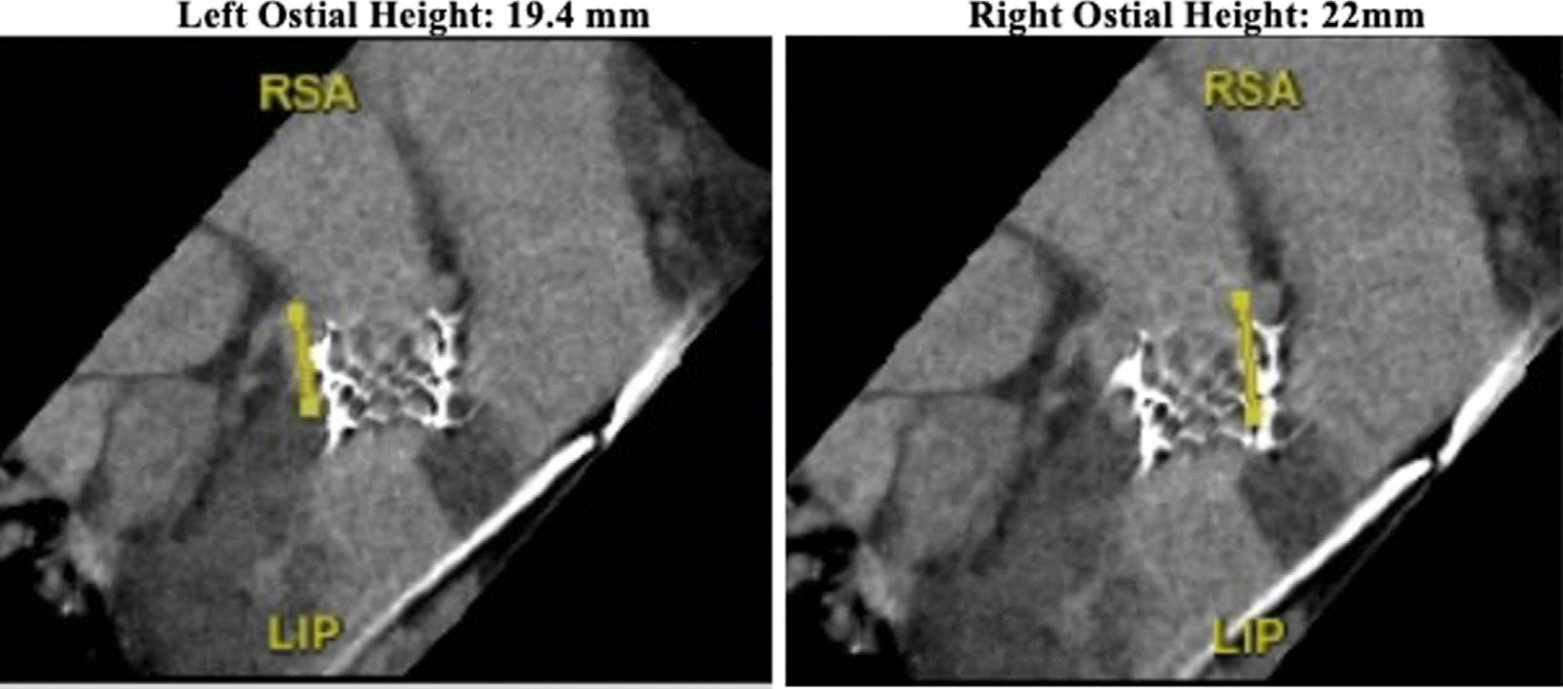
Computed Tomography Showing Right and Left Coronary Heights Relative to Previous Transcatheter Aortic Valve Replacement
